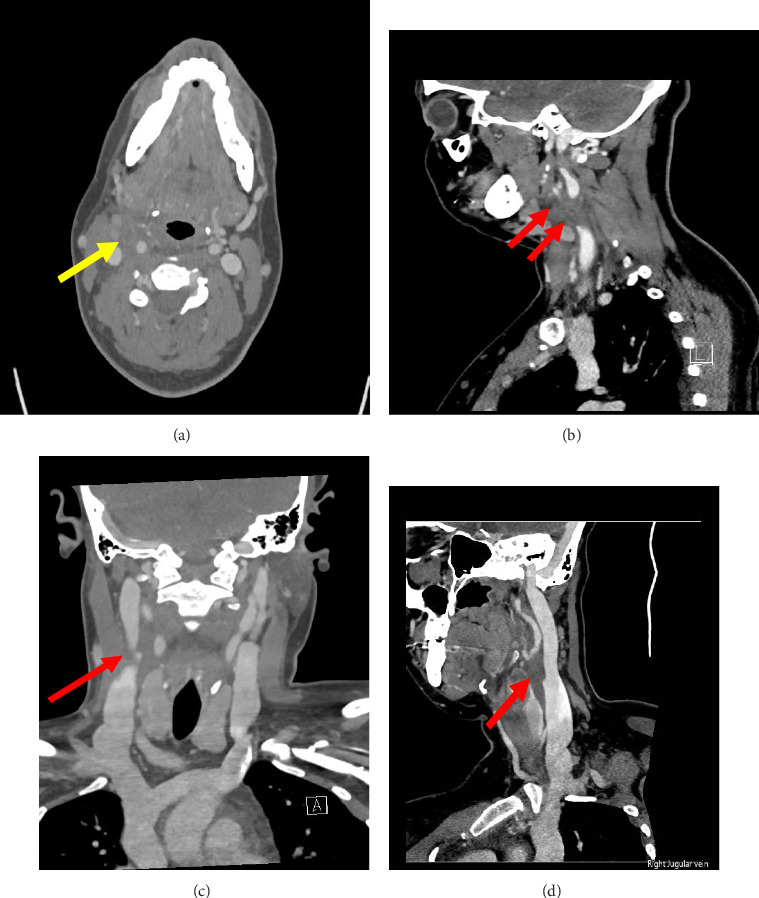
(a) Axial CT venogram revealing a small fluid collection (yellow arrow), suggestive of tonsillar abscess. (b, c, d) Coronal, sagittal, and single right internal jugular curve views demonstrating a filling defect of the right facial vein near the inflow of the right internal jugular vein (red arrows), suggestive of thrombosis.
